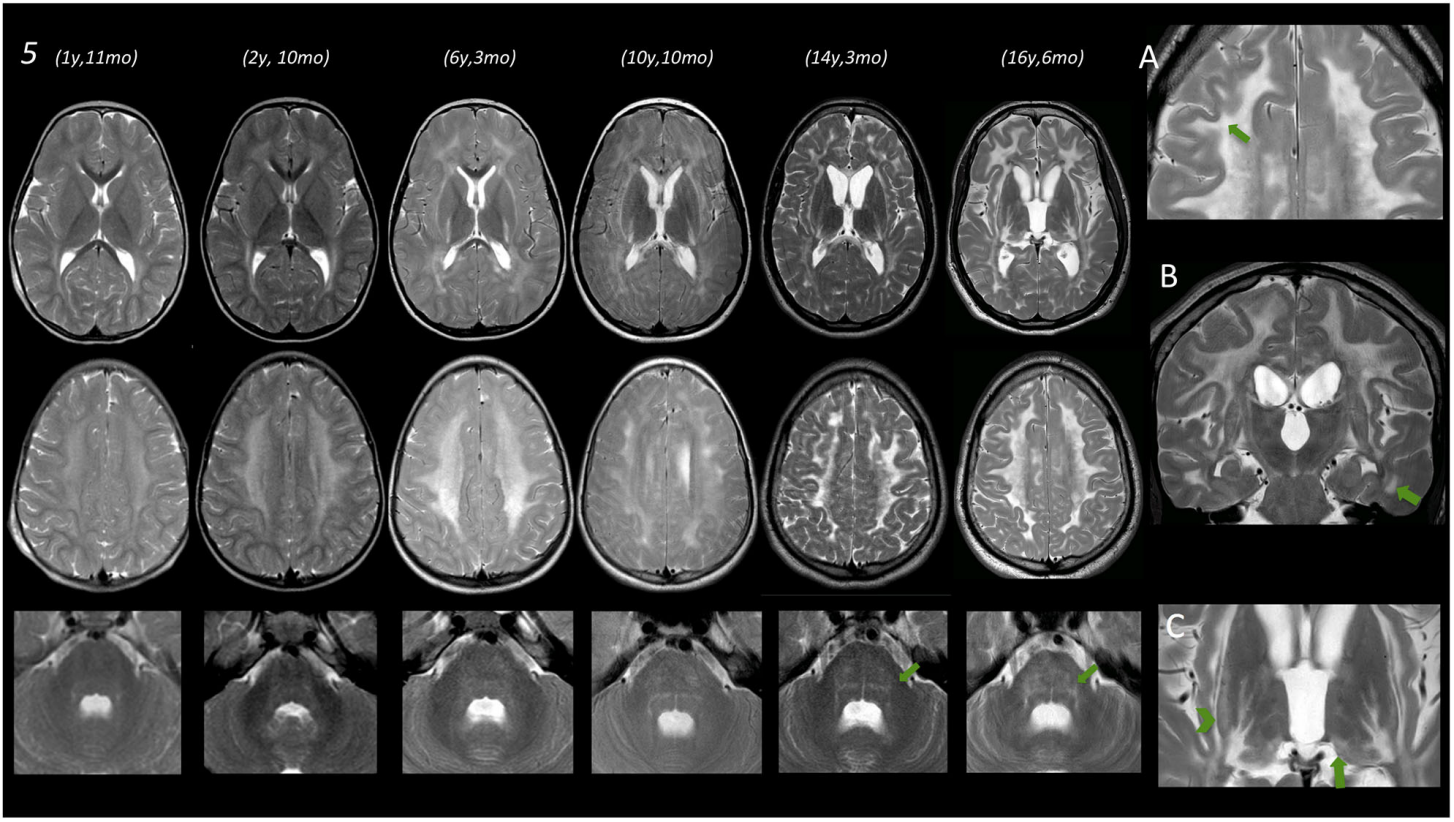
Brain MRIs of subject no. 5. In the left part of the figure, MRIs of three different levels (deep nuclei, centrum semiovale, and middle cerebellar peduncles) are displayed. Under the age of 3 years, only a very mild T2 hyperintensity is evident at the level of the periventricular WM. Subsequent MRI scans show a progressive marked diffuse T2 hyperintensity of the WM also involving the posterior limb of the internal capsule (PLIC) as well as the external and the extreme capsule bilaterally. Infratentorially, only a mild T2 hyperintensity is evident at the age of 6 years 3 months at the level of the dorsal pons that becomes more pronounced at the age of 10 and 14–16 years. (A) Magnification of axial T2 image acquired after the age of 16, showing a remarkable involvement of the U fibers (arrow). (B) Coronal T2 image acquired at the age of 16 years showing involvement of temporal WM (arrow) and also T2 hyperintensity of corticospinal tracts. (C) Magnification of axial T2 images at the age of 16 showing T2 hyperintensities at the level of the tail of the putamen (arrowhead) and the level of the pulvinar (arrow).
